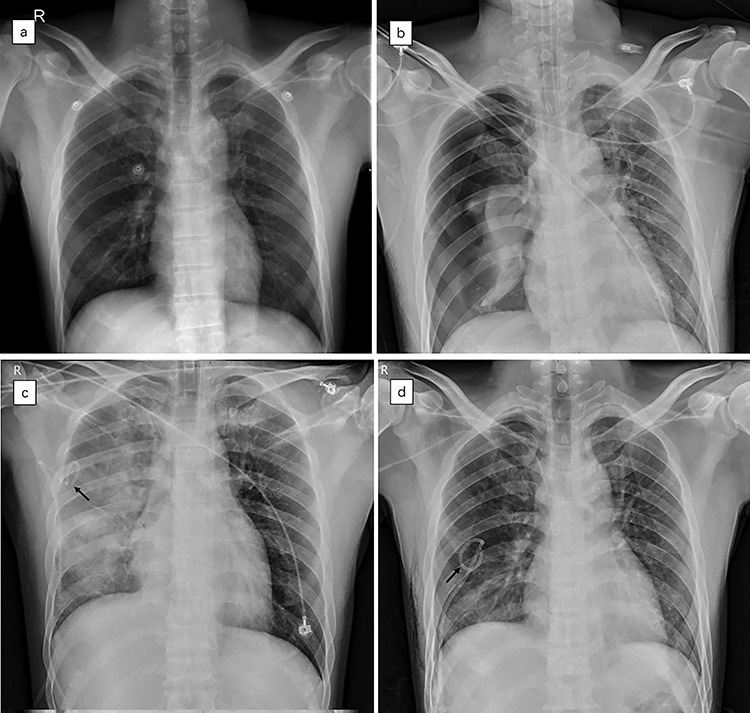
Temporal sequence of chest X-ray imaging of the patient suffering from re-expansion pulmonary edema (REPE). (a) Chest X-ray showing normal pulmonary field of both hemithorax after injury. (b) Chest X-ray showing large right pneumothorax (estimated 85%) with collapsed right lung and contralateral mediastinal deviation. (c) Chest X-ray showing most of the right lung has been re-expanded, accompanied by massive exudative change. (d) Chest X-ray showing bilateral clear lungs with free pleural space after 3 days of treatment. The black arrows indicate the position of the chest tube
